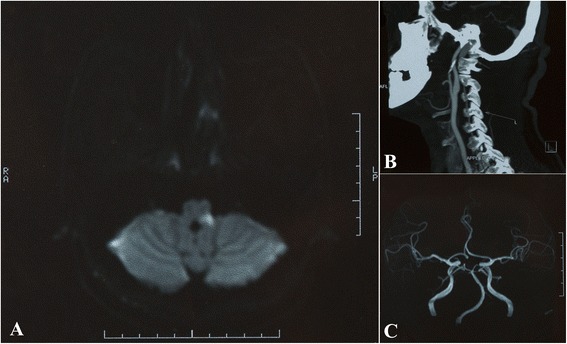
Magnetic resonance imaging (MRI) and CT angiography findings. (A) Diffusion-weighted magnetic resonance imaging showed region of hyperintensity in the left dorsolateral medulla. (B) CT angiography showed that the left vertebral artery became slender in the cervical part. (C) MR angiography showed a local blood flow disappearance of the left vertebral artery and basilar artery distortion.
